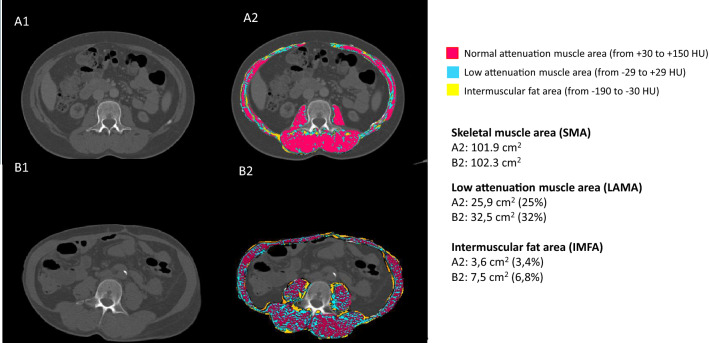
Representation of different levels of fat infiltration in skeletal muscle area assessed by computed tomography in the third lumbar vertebra in two female patients (age 43 years old) on hemodialysis. A1 and A2: images from the same patient before and after segmentation. B1 and B2: images from the same patient before and after segmentation. Patient represented in A2 has similar skeletal muscle area (SMA) to that of the patient in B2, but the patient in B2 has a higher proportion of low attenuation muscle area (LAMA) and higher intermuscular fat area (IMFA). The patient represented in A2 has similar SMA to that of the patient in B2, but the patient in B2 has a higher proportion of LAMA and higher IMFA, indicating lower muscle quality and higher muscle fat infiltration
